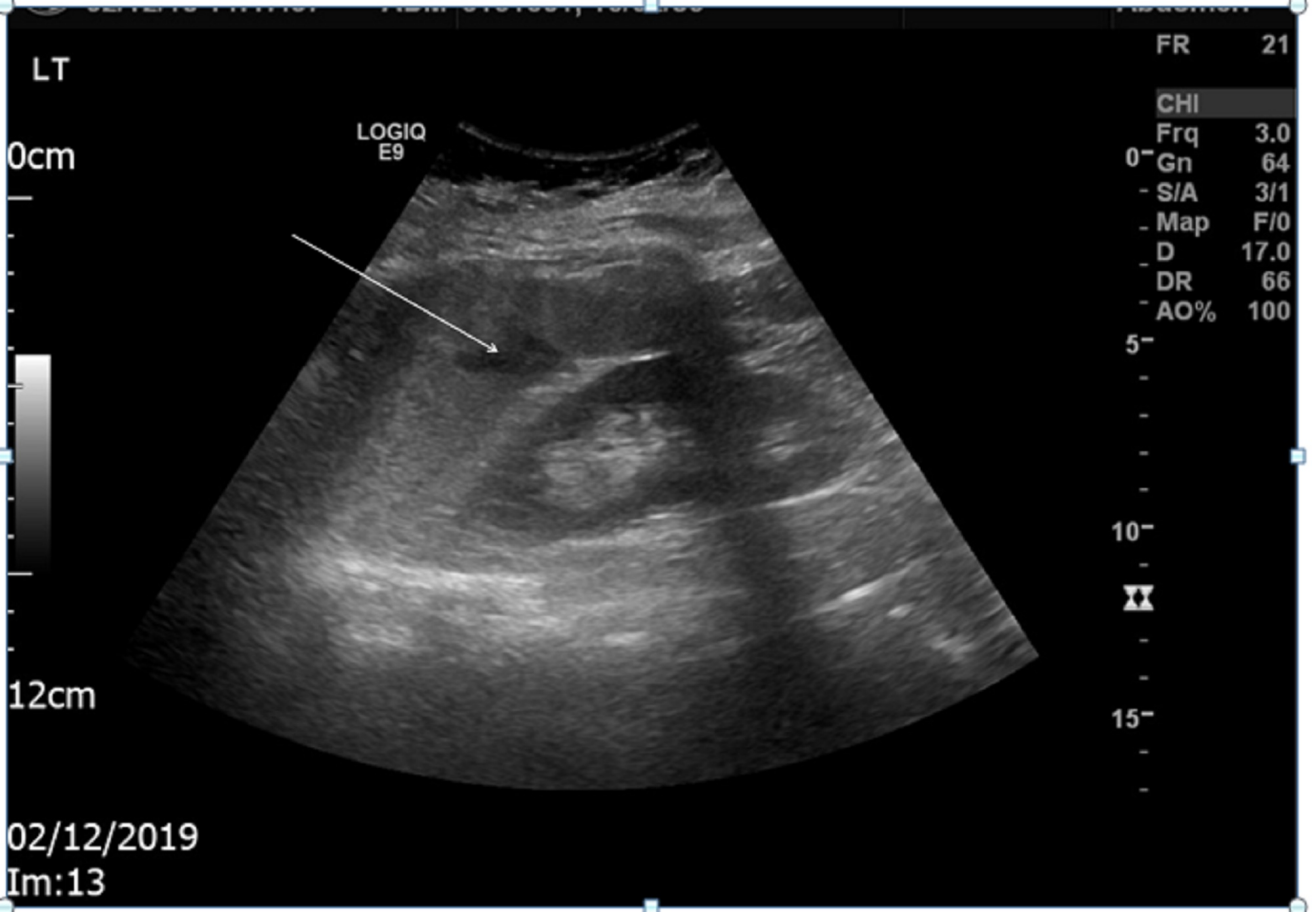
Abdominal ultrasound of the patient Splenic infarction is indicated by the arrow
